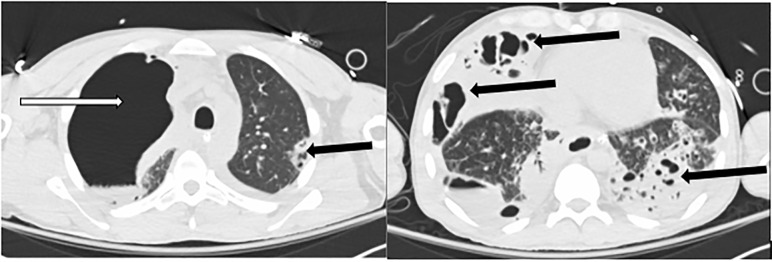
Thorax computed tomography transverse plane demonstrating giant right upper lobe bulla (white arrow) as well as multifocal pneumonia and bronchiectasis in both images at differing levels (multiple black arrows).
